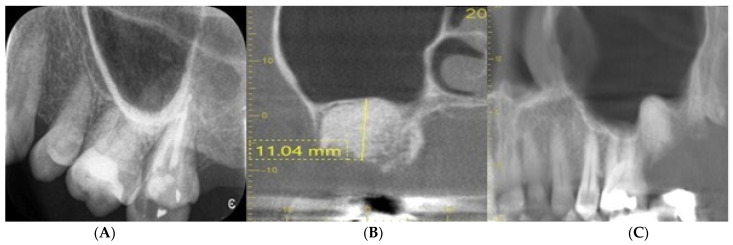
CBCT sections (A) shows the upper left molars before extraction (B,C) showing preservation of the alveolar ridge morphology following SA of UL 7 extraction site.
